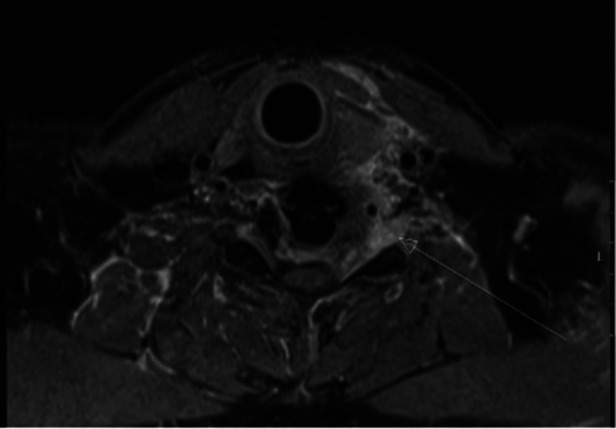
Postoperative axial T1-gadolinium MRI showing partial removal of tumor mass.
